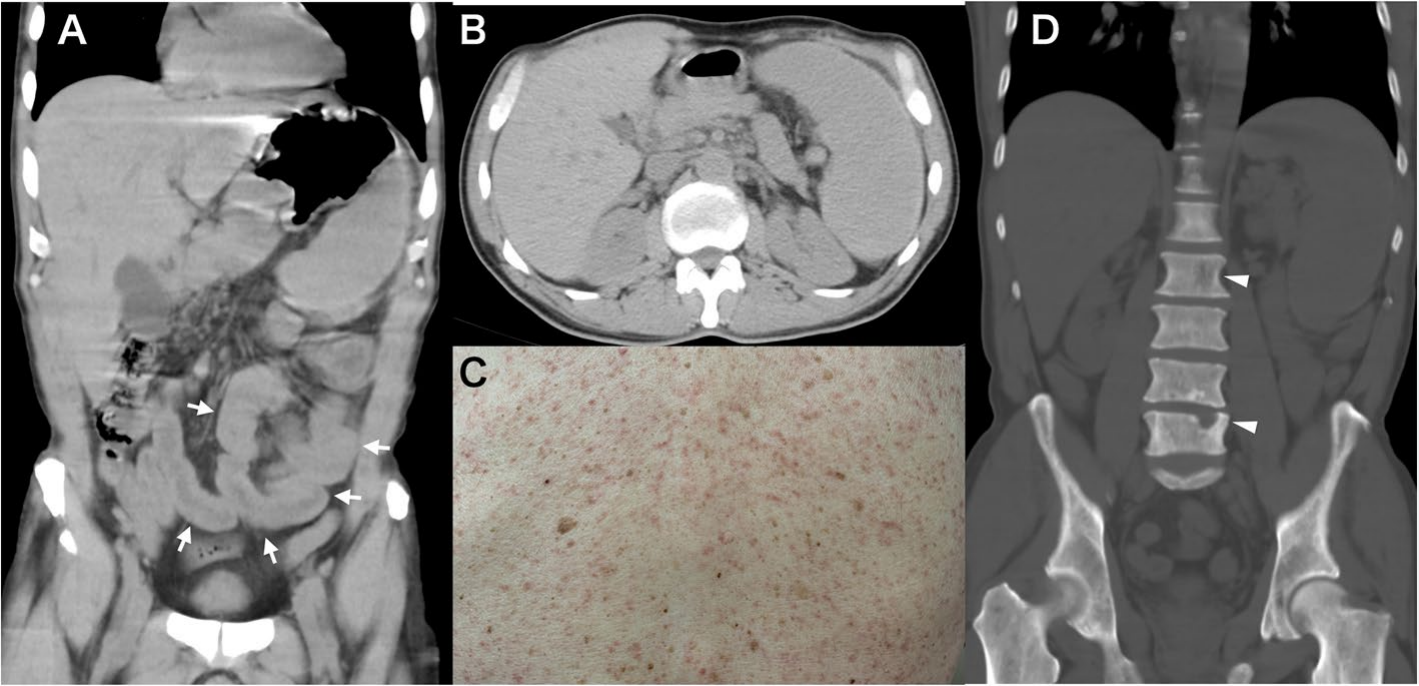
Abdominal CT of the patient showing A an edematous and swollen small intestine (arrows), B splenomegaly, and C maculopapular cutaneous mastocytosis on the back. D Bone density CT showing osteosclerosis of the pelvic bones, with partial punched out osteopenia of the spine (arrowheads)
